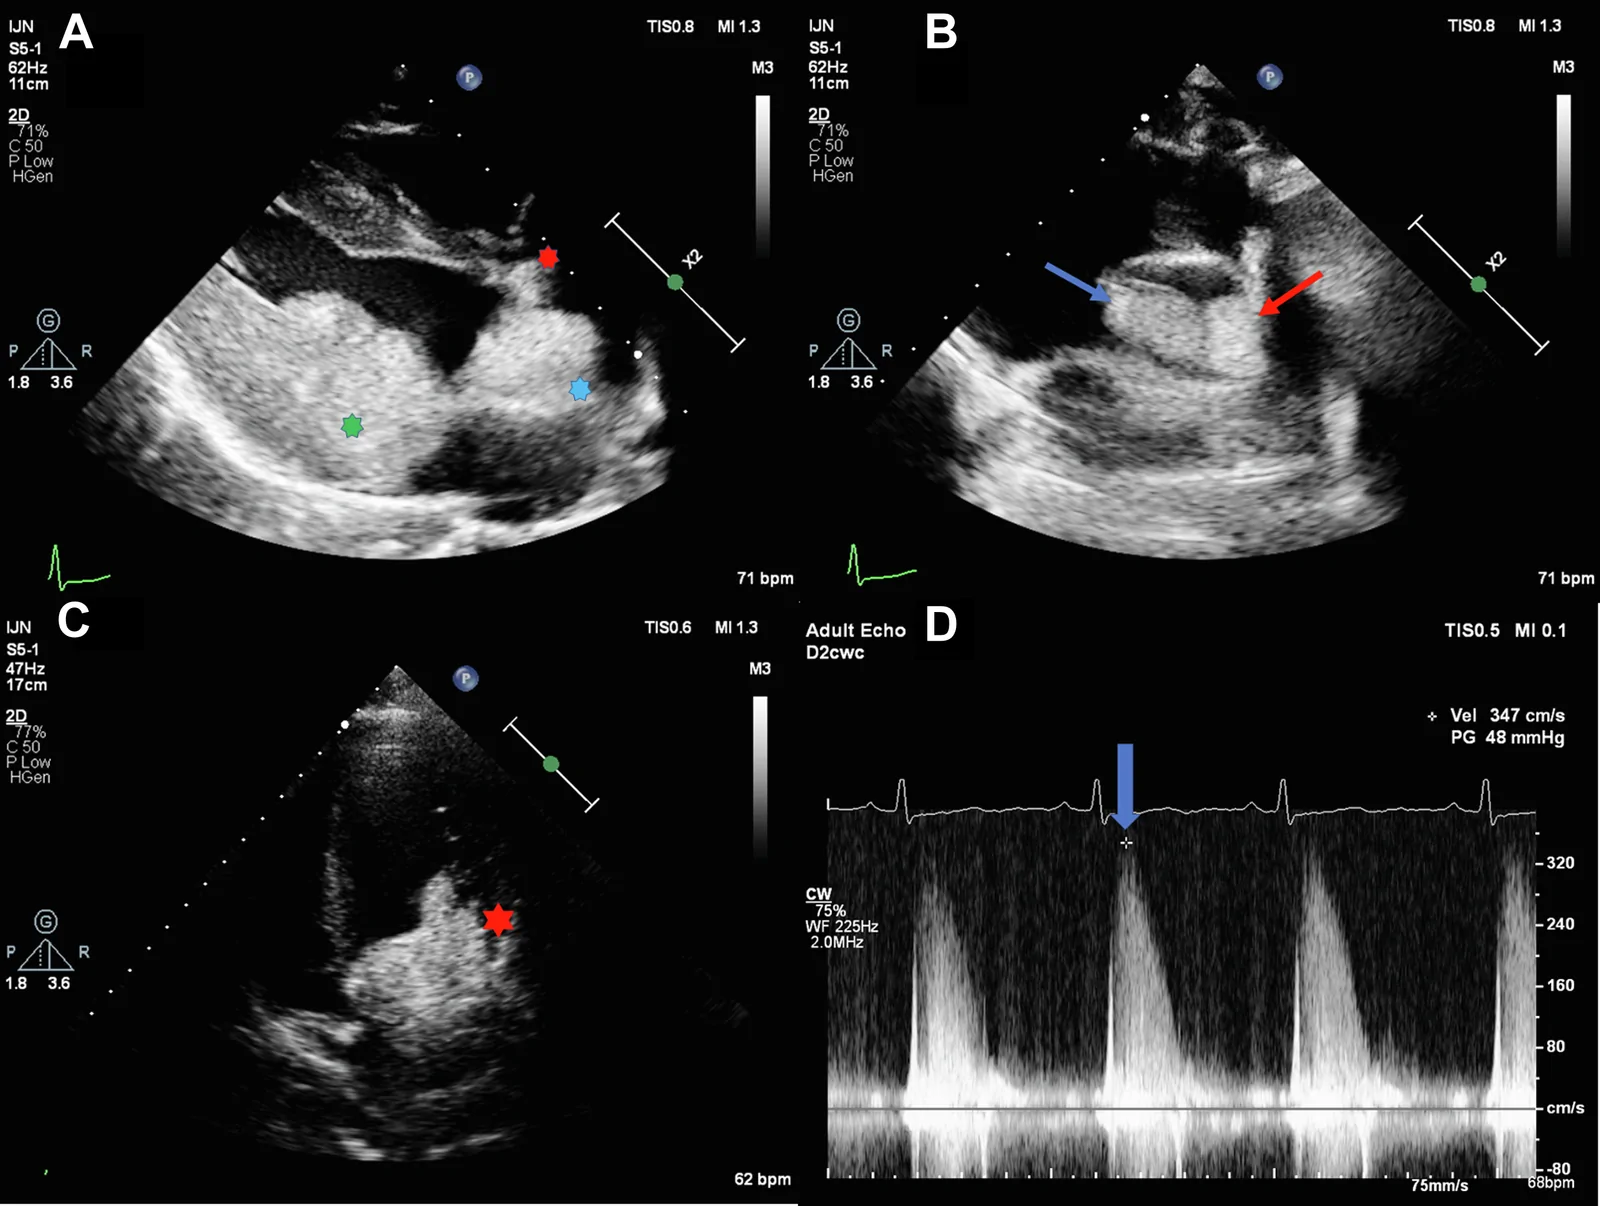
2D Transthoracic Echocardiography (TTE) and Continuous Wave (CW) Doppler Across Aortic Valve (A) Three cardiac masses at the (1) left ventricle wall (green star), (2) noncoronary cusp (blue star), and (3) left coronary cusp (red star) are demonstrated on a parasternal long-axis view in transthoracic echocardiography. (B) Noncoronary cusp (blue arrow) and left-coronary cusp (red arrow) masses seen on a short-axis view in transthoracic echocardiography. (C) Left ventricular wall mass seen on an apical four-chamber view. (D) Doppler signal showing severe aortic regurgitation. bpm = beats/minute; CW = continuous wave; IJN = internal jugular vein; MI = myocardial infarction; PG = pressure gradient; TIS = tissue imaging system; VEL = velocity.
